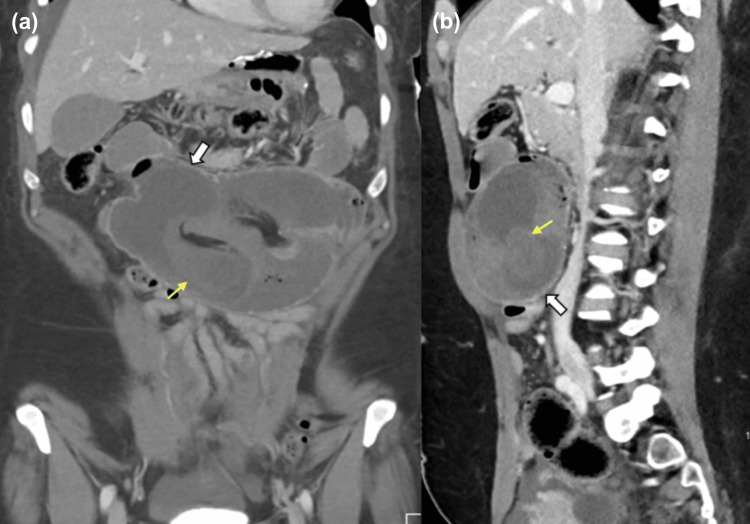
(a) Coronal and (b) sagittal reformats of an enhanced abdomen and pelvis CT scan on the portovenous phase. The Roux-en-Y afferent loop is intussuscepted within the jejunojejunostomy (white arrow). The entry point within the intussuscepted loop (yellow arrow) with mesenteric fat subsequently shows reduced enhancement of the entrapped-closed loop within the intussusception loops.
